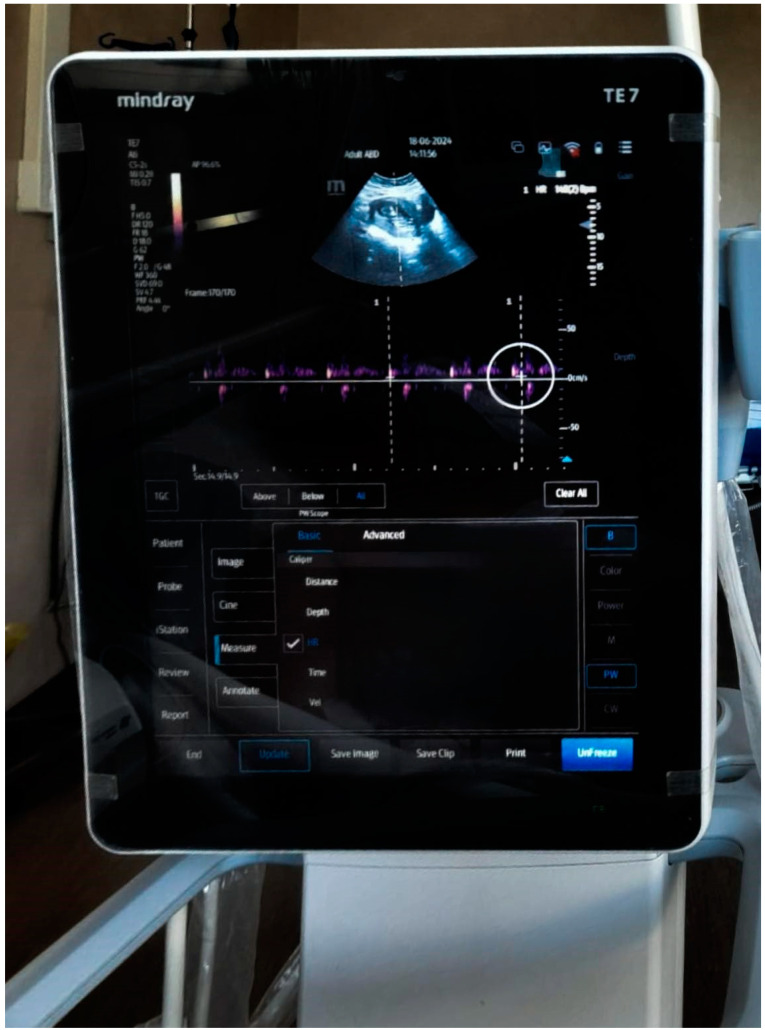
Fetal heartbeat ultrasound performed immediately postoperatively.
